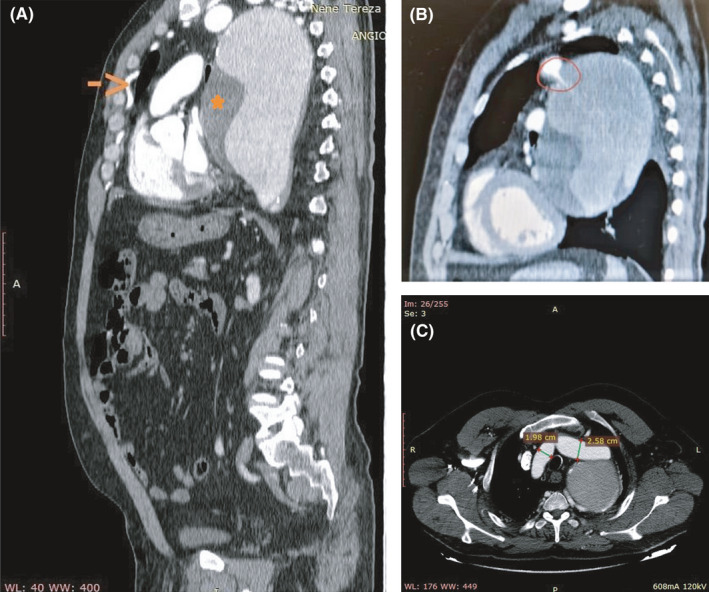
(A) Sagittal thorax reconstructed CT images showing the presence of hypertrophic intercostal arteries (arrow) as well as the giant aneurysm with a dissected flap (asterisk). (B) Coarctation neck (drawn circle) in the sagittal, contrast‐enhanced image. (C) Presence of right and left brachiocephalic truncus, with respective transverse diameters in centimeters
